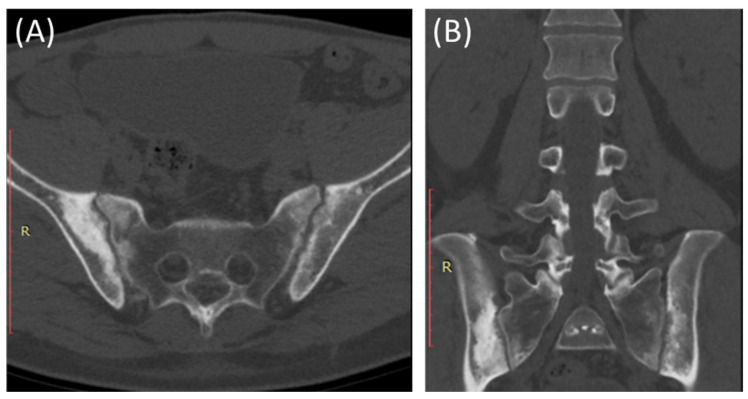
Axial bone window CT scan (A) and coronal bone window CT scan (B) for a 41-year-old male presenting with Rt sciatica suggestive of bilateral sacroiliitis (same Patient as in Figure 3).
